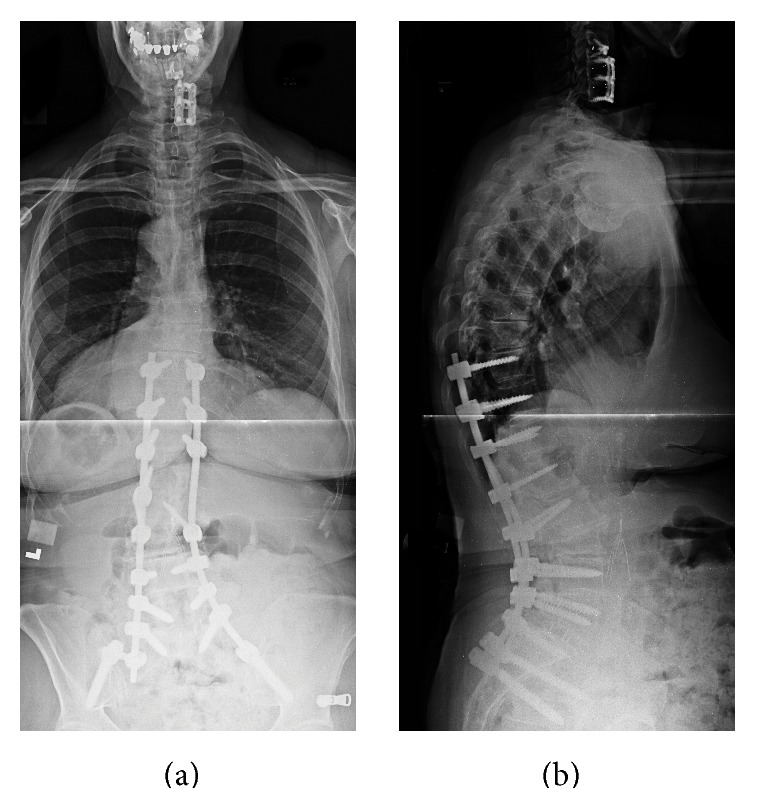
Posterior-anterior (a) and lateral (b) postoperative 3-foot scoliosis X-rays. Note the markedly improved coronal and sagittal balance achieved after the deformity correction.
